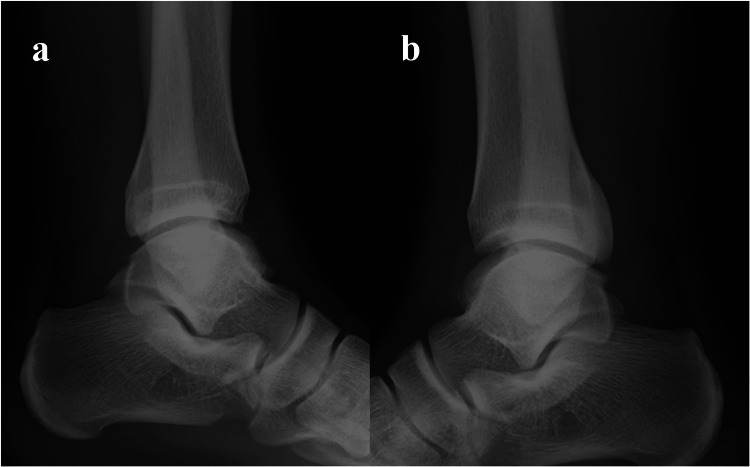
Lateral ankle radiography at the initial visit (a) Unaffected side. (b) Affected side
